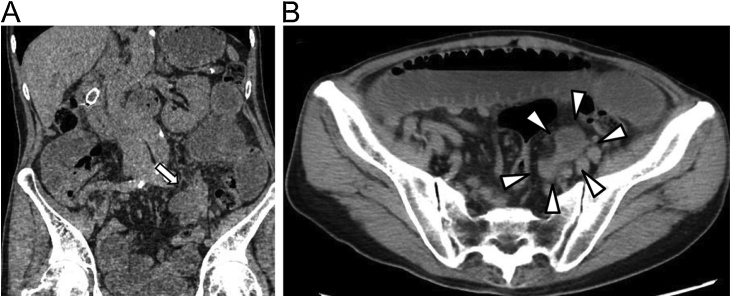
Preoperative multidetector-row computed tomography. (a) The sac-like mass suggests that a closed loop of the small bowel exists in the sigmoid colon dorsum. (b) A cluster of dilated small bowel loops resembling the figure “8.”
